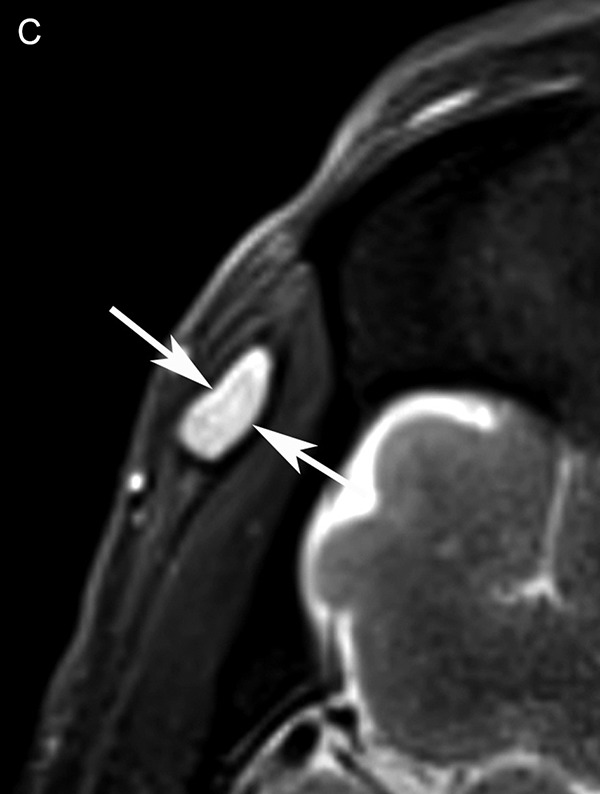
New MRI performed at our institution. The lenticular formation located in the deep hypodermal plane of the temporal region (arrows) shows a low to intermediate signal on T1-weighted image (A), with signal enhancement after contrast injection (B), and a high signal on T2-weighted images with fat saturation (C). Note the heterogeneity of the central portion of the mass, which contains small unenhanced areas (black arrowhead in B).
